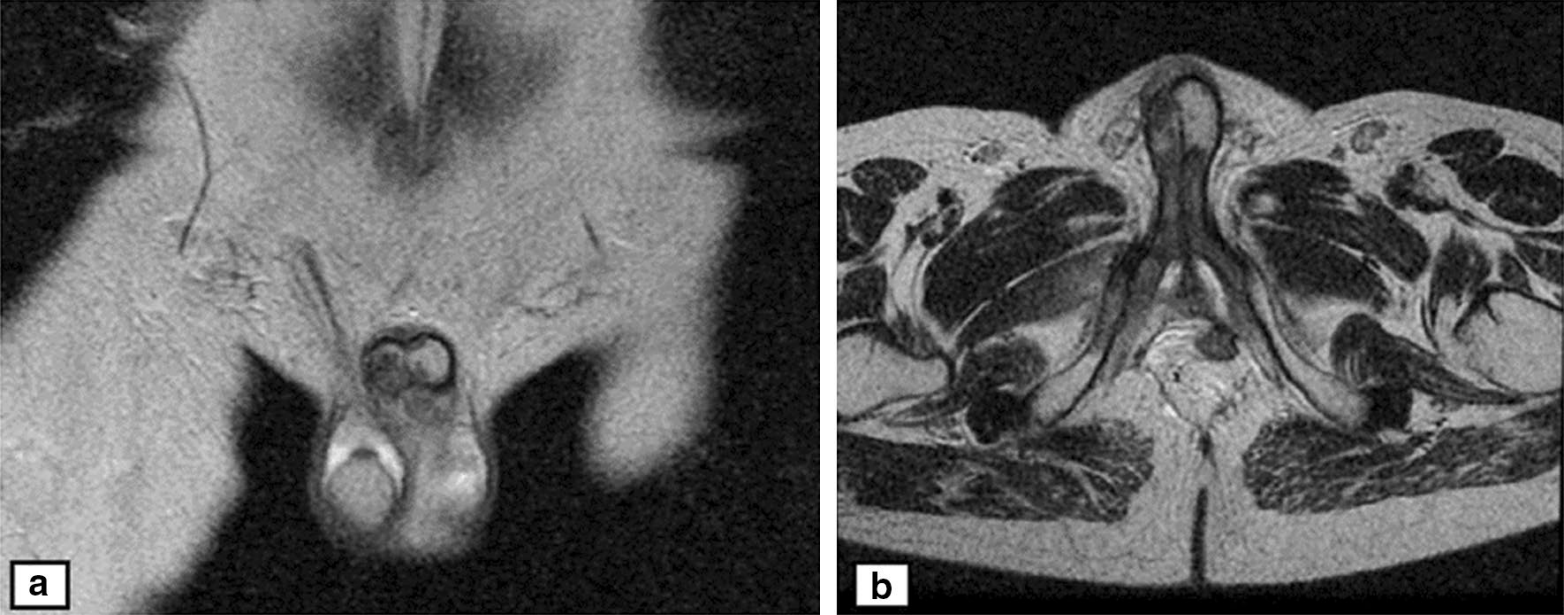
The magnetic resonance imaging (MRI) showing tumoral infiltration of the right corpora cavernosa (a), penile bulb and neighboring perineal soft tissue (b)
